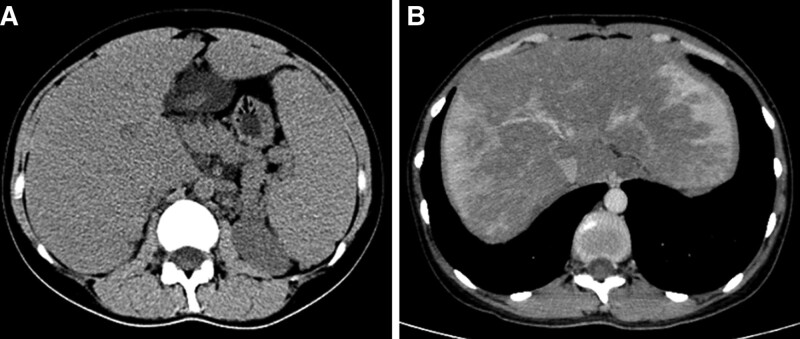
Hepatobiliary CT scan + enhancement. (A) Full liver morphology, splenomegaly. (B) Uneven density of hepatic veins. CT = computed tomography.
